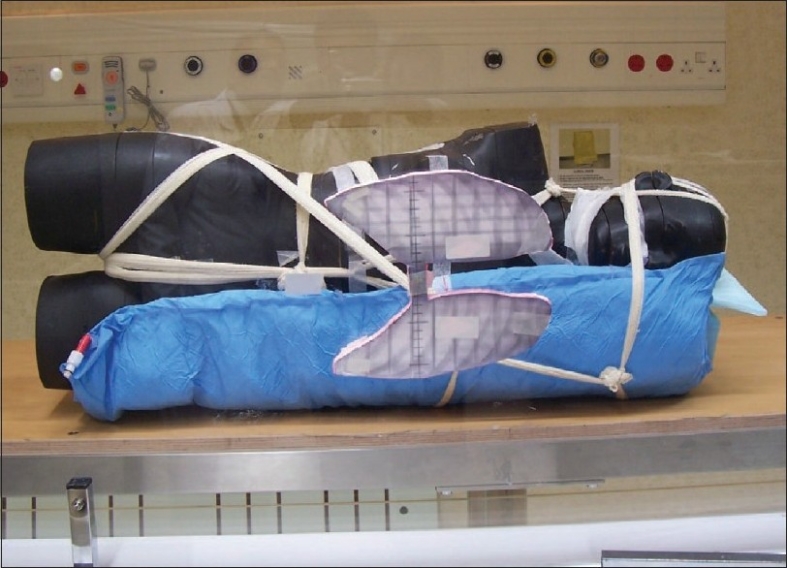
Position of Styrofoam Shield Template on Beam Spoiler in Verification Radiography
